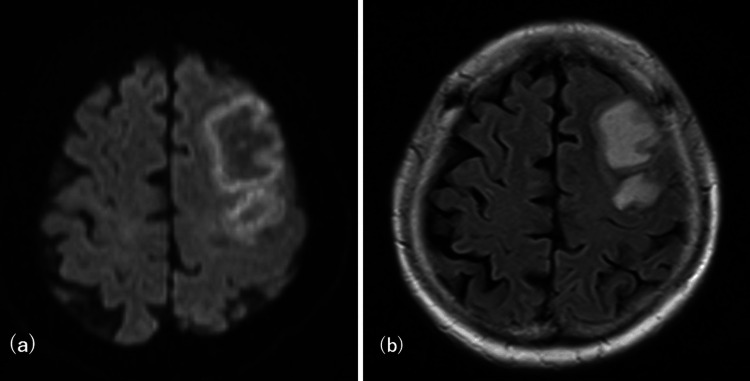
MRI on admission showed lesion expansion MRI: magnetic resonance imaging (a) Diffusion-weighted imaging (DWI). (b) Fluid attenuated inversion recovery (FLAIR)
